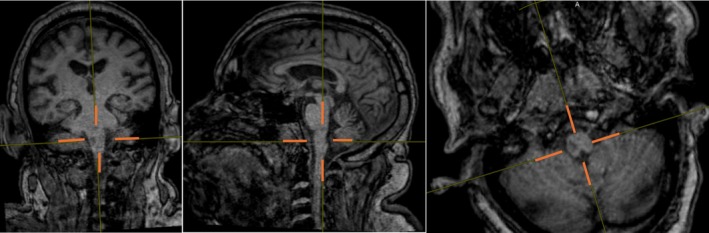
Perpendicular to the axial MRI brain T1 slices displaying the infarct involvement of the pyramidal decussation at the medulla–spinal cord junction.
